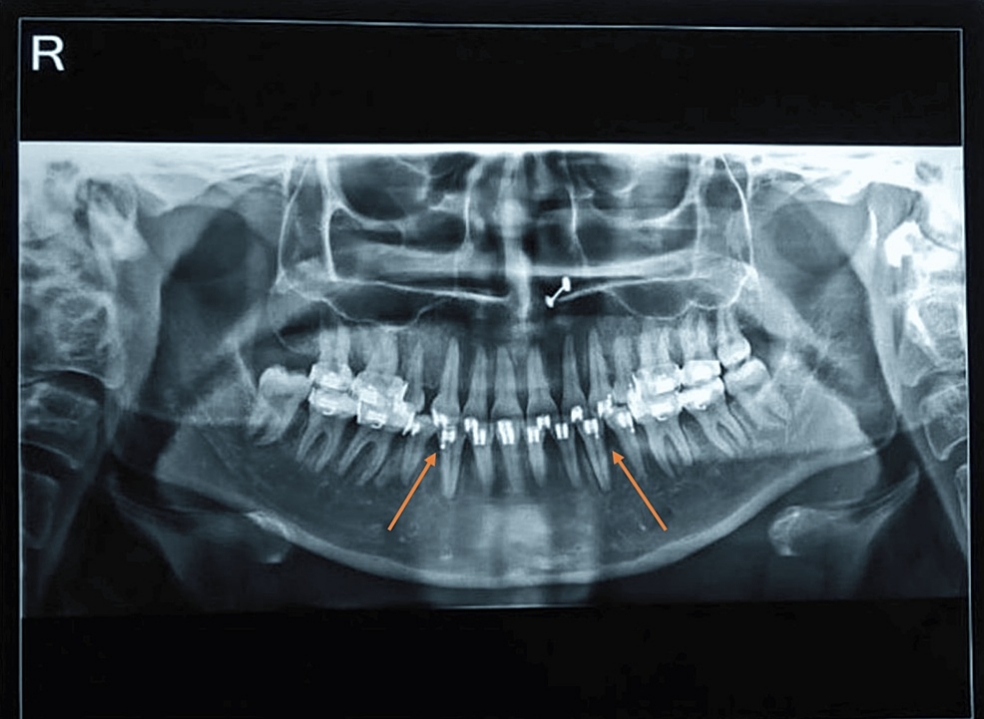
Post-treatment orthopantomogram
